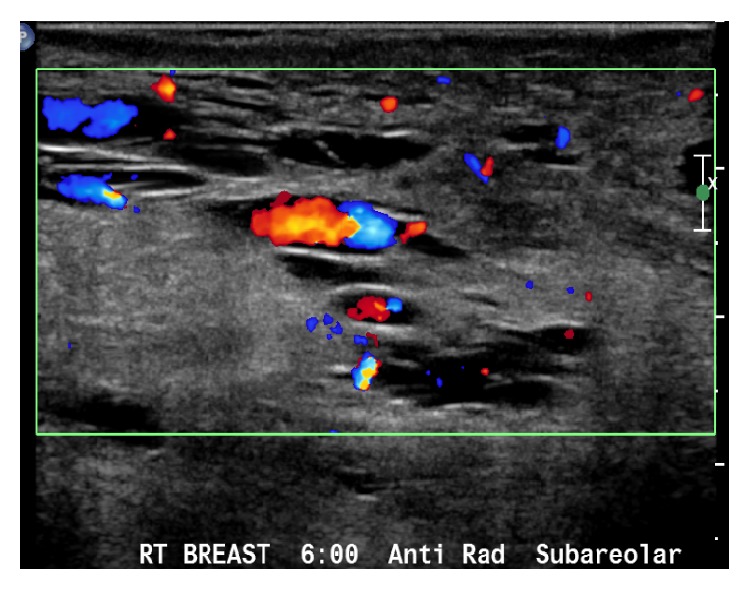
Breast ultrasound: 6 o'clock: huge ducts that should be going in the direction of the nipples (but there are not!). These dilated ducts end bluntly and are not connected to the outside. Therefore, the fluids and materials in them cannot decongest and stay as fluid filled.
